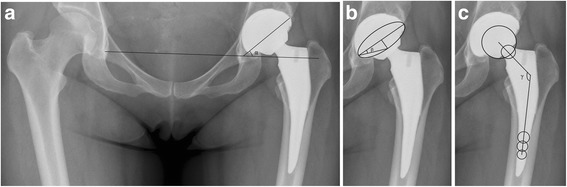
The cup orientation and stem version were measured in an anteroposterior pelvic radiograph. a Postoperative acetabular inclination: α. b Postoperative acetabular anteversion: β. c The angle between the axes of the neck and stem was regarded as the neck–shaft angle: γ. The stem version was assessed by the γ
